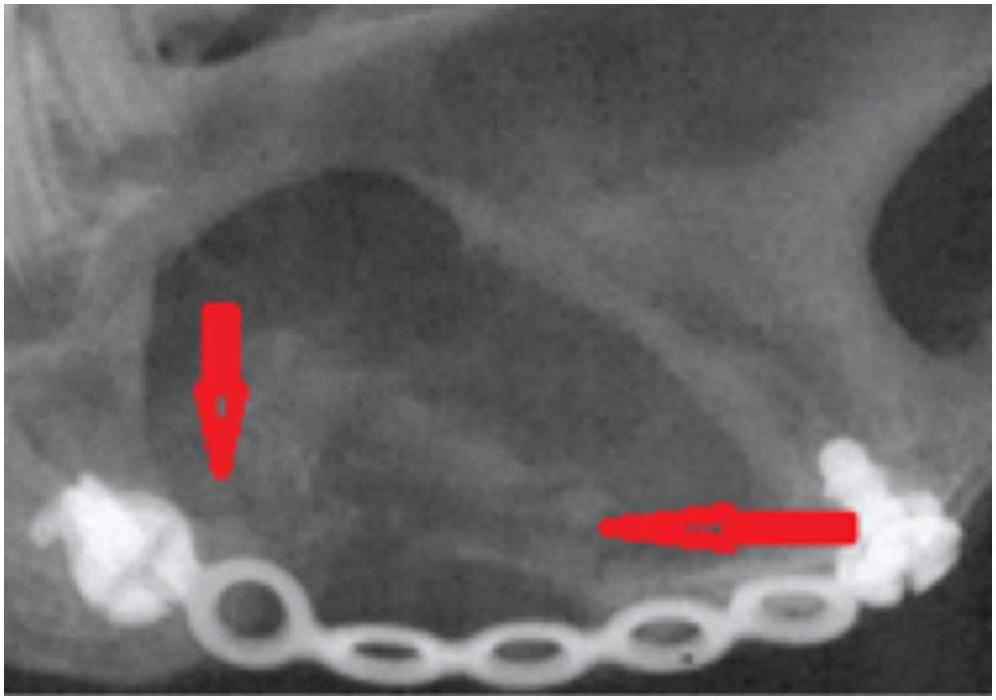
Micro CT scanning for one of the samples showing the Field of Vision (FOV) that was selected for all the samples; blue arrows indicate the boundaries of FOV, the anterior arrow (A) refers to area of the last premolar tooth; the last proximal screw represents the posterior limit (P) of the FOV, vertically the FOV extended 1 cm above the ramus notch denoted with yellow arrow.
